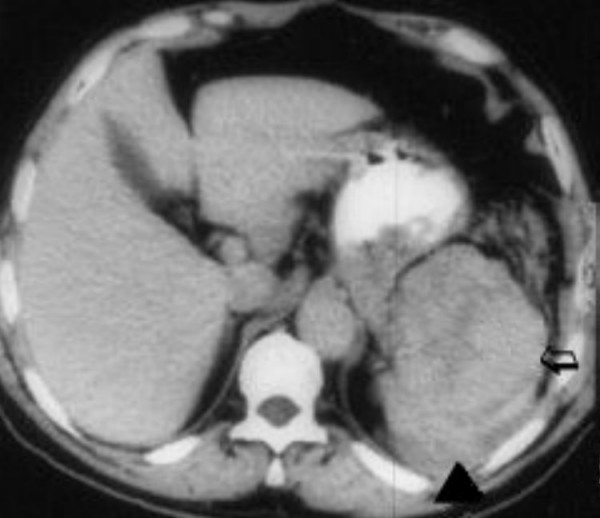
Abdominal computed tomography scan demonstrating intraperitoneal free fluid (arrowhead) and an enlarged, anechoic spleen with hypoechoic areas representing focal necrosis (arrow).
